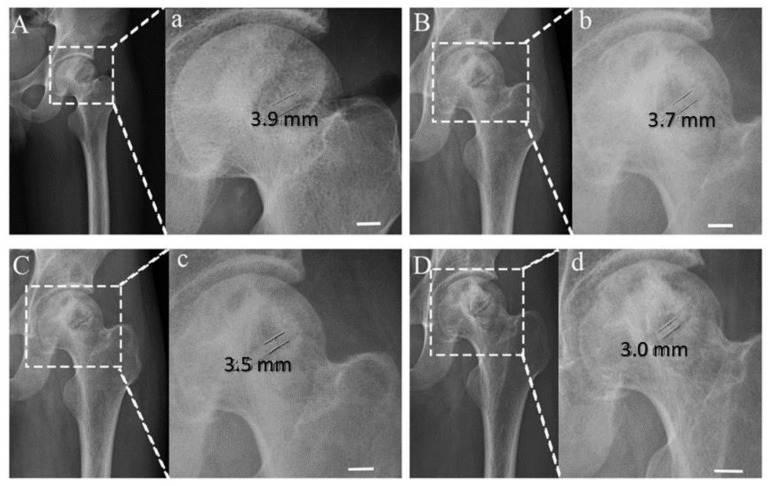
The temporal changes in biodegradation rate of Mg screws interpreted by a decrease in screw diameter. (A–D) X-ray imaging of femoral head in patients implemented with Mg screws at 1 (A), 3 (B), 6 (C), and 12 (D) months postoperatively. (a–d) Magnified surgical regions in (A–D) for measurement of screw diameter at different time points. Scale bar represents 10 mm [74].
